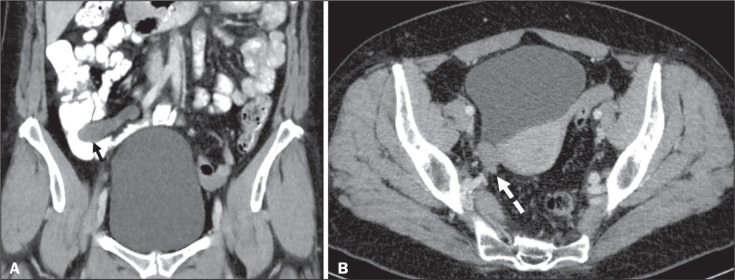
Mucocele of the appendix. Contrast-enhanced coronal and axial CT scans (A
